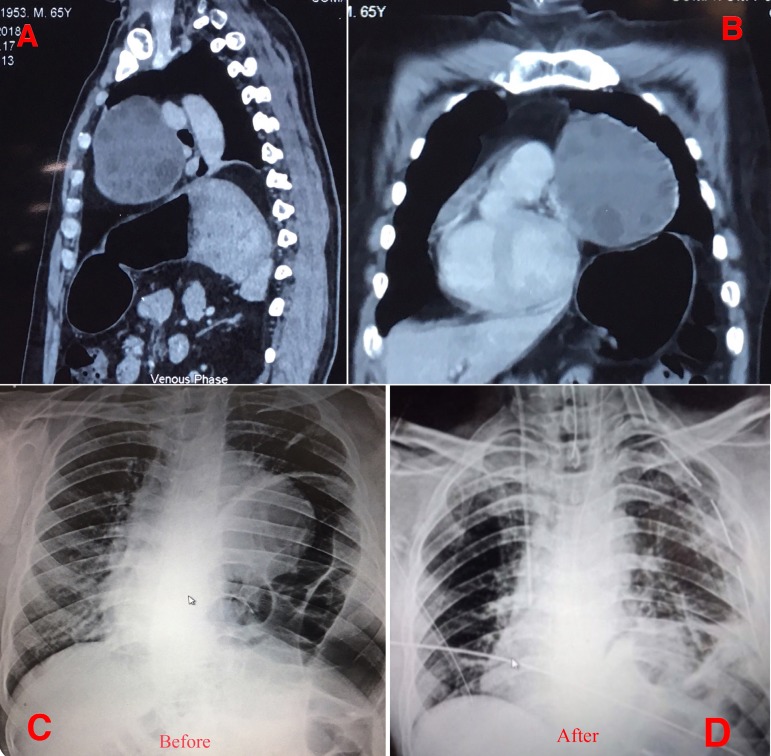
A and 1B - Computer tomography images showing the mediastinal cyst with daughter cysts. C and D - Pre and post left hemidiaphragmatic plication X-rays.
